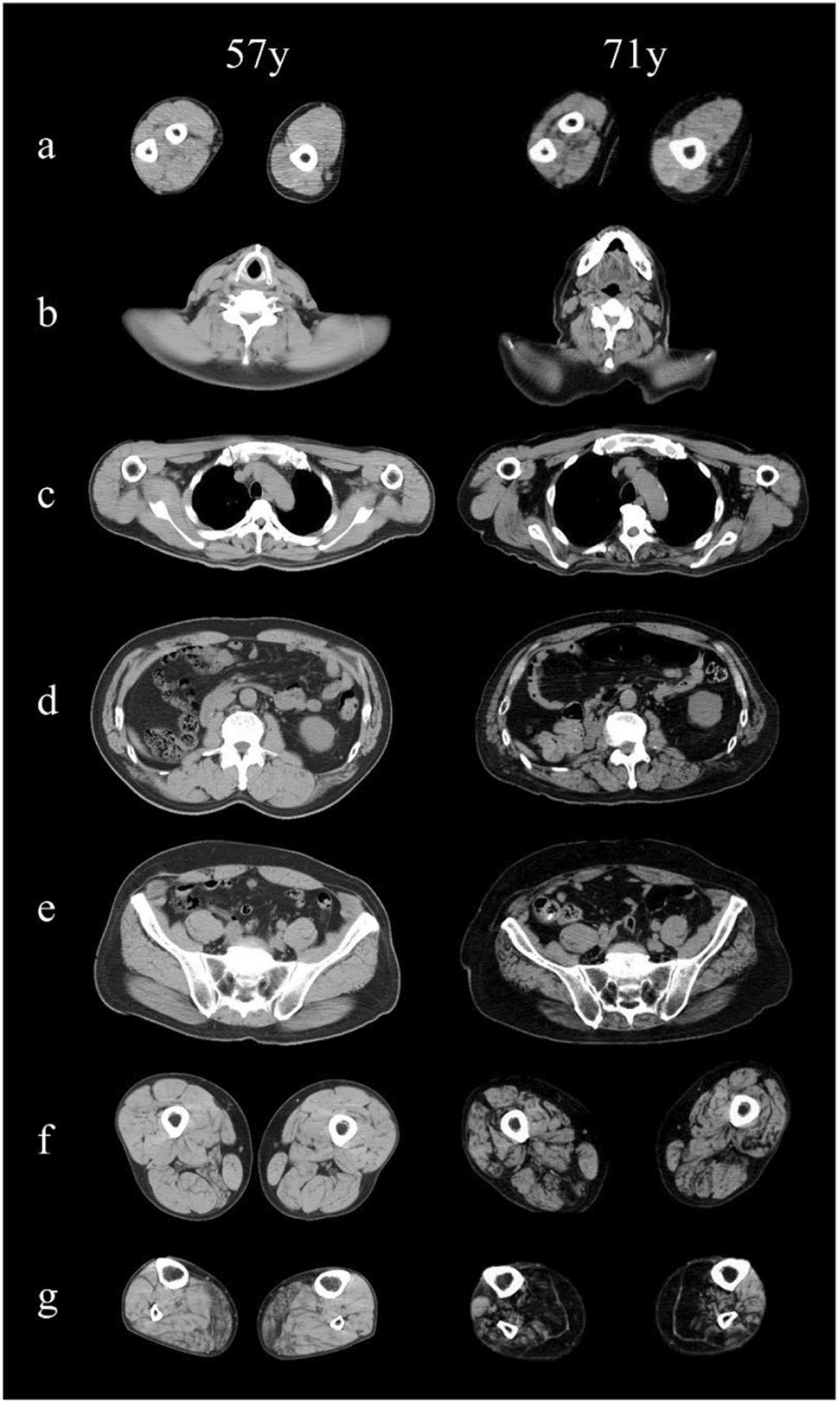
Muscle computed tomography. Muscle computed tomography was evaluated twice in Pt-II at the ages of 57 and 71. The images show slices of the forearm and arm (a, left and right), neck (b), thorax (c), abdomen (d), pelvis (e), thigh (f), and distal legs (g). Symmetrical fatty changes in the medial head and to a lesser extent the lateral head of the gastrocnemius are present at 57 years (g, left panel), whereas pathological muscle atrophy cannot be identified in other levels. At 71 years, all lower leg muscles were severely fatty-replaced, relatively sparing the tibialis anterior and extensor digitorum longus muscles (g, right panel). The thigh exhibited moderate and diffuse atrophy; however, the hamstring muscles are predominantly affected (f, right panel). In the truncus, paraspinal muscles are fatty-degenerated continuously from the cervical level to the lumbar level. In the upper extremities, diffuse muscle atrophy is more prominent in the forearms than in the arms (a, right panel)
